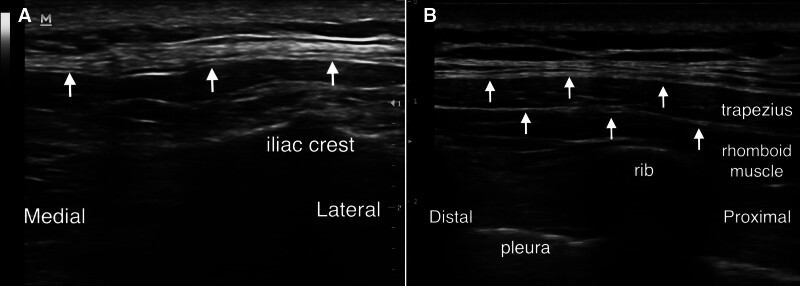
Ultrasound findings in patients with myofascial pain syndrome (MPS) reveal localized thickening and increased echogenicity of the fascia. (A) The white arrow points to the thickened, hyperechoic thoracolumbar fascia. (B) The white arrow indicates the thickened, hyperechoic fascia overlying the trapezius and rhomboid muscles. Both 2 patients experience significant localized pain.
